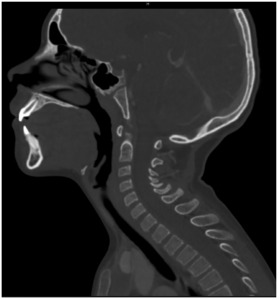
Sagittal view of computed tomography soft tissue neck with contrast with evidence of maxillary sinus disease and without evidence of hemorrhage or other infectious etiology for torticollis.
